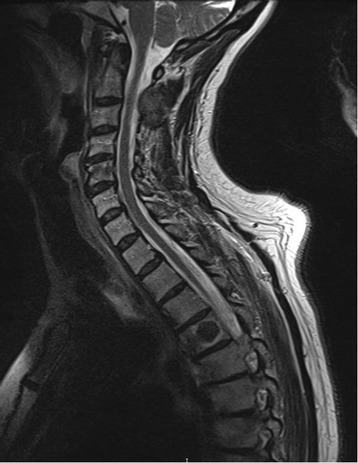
T2-weighted sagittal magnetic resonance image demonstrating the deposits in C5 and T4. They appeared confined to the vertebral body with no evidence of vertebral body collapse
